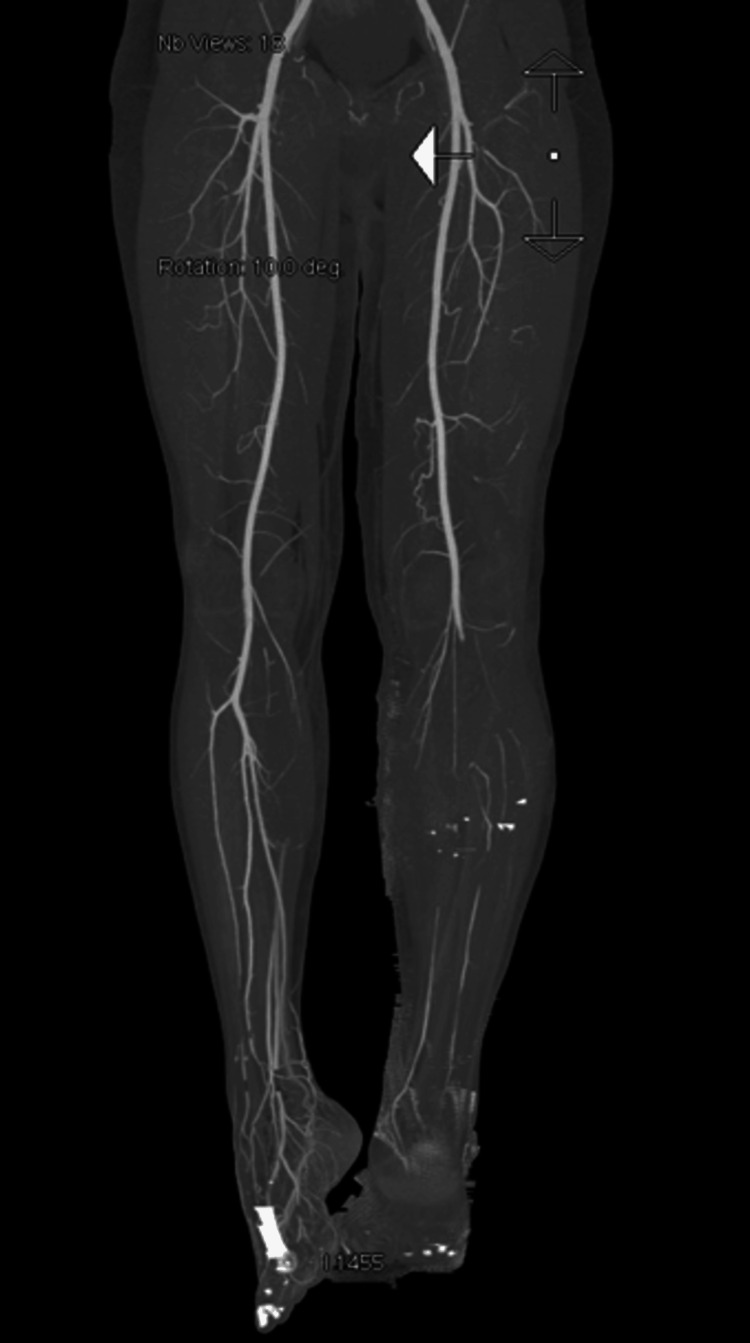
CT angiography revealed a popliteal artery injury, and intact medial and lateral sural artery supplying the gastrocnemius muscles.
